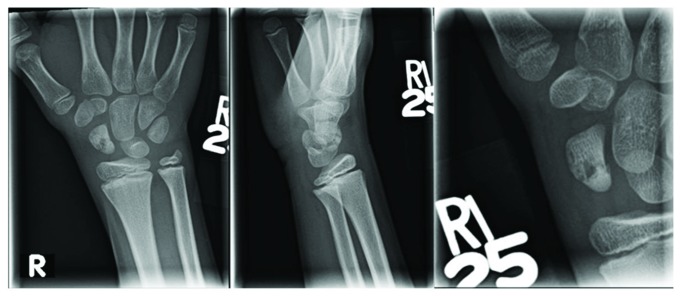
AP and lateral radiographs from November 2016 (approximately 3 years and 1 month from the initial injury) demonstrating a nondisplaced scaphoid waist fracture and a subtle osteosclerotic appearance within the proximal pole. There is also a relative sclerotic appearance of the scaphoid relative to the other carpal bones—a constellation of findings concerning for osteonecrosis [26].
